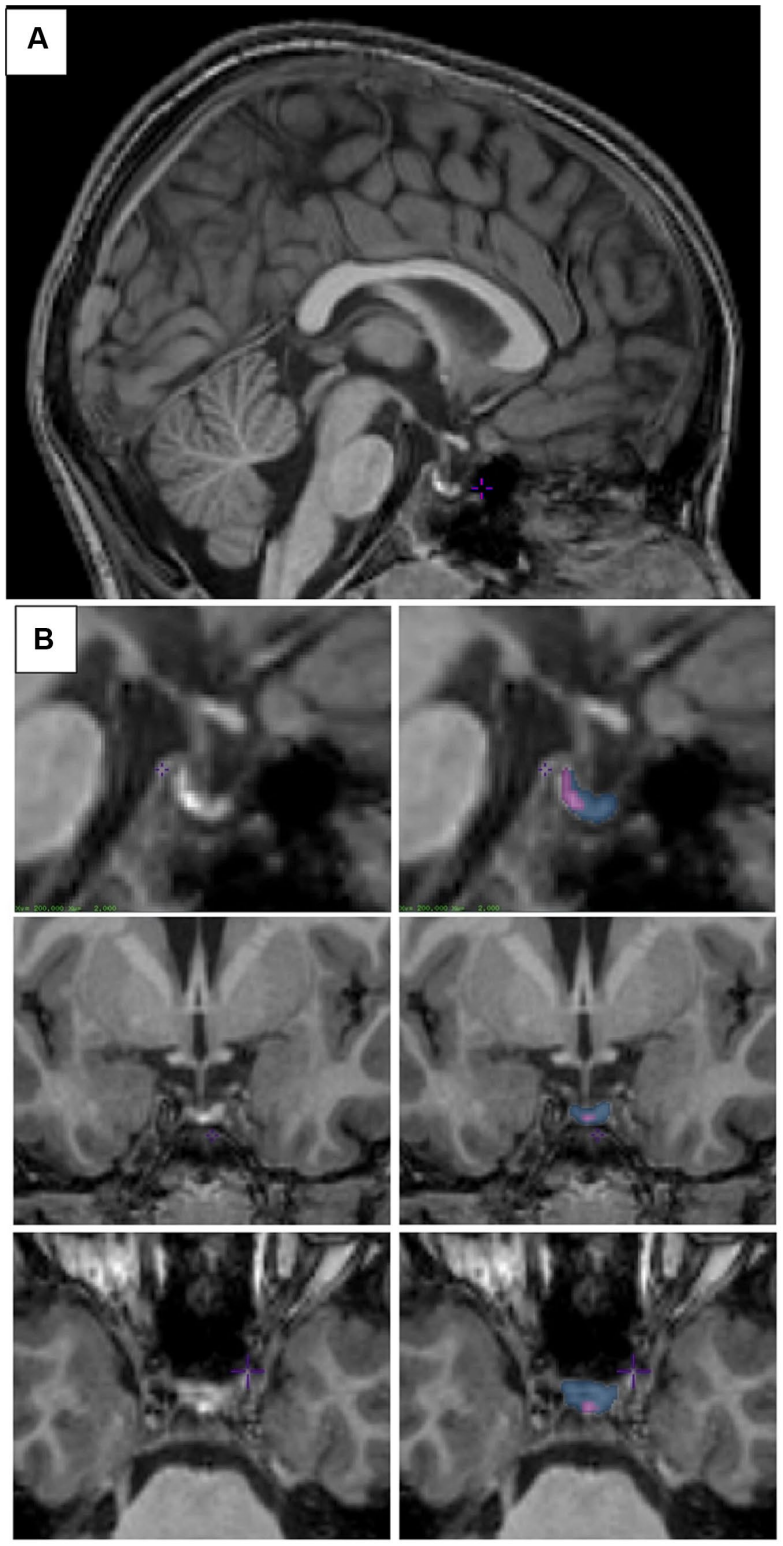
Pituitary segmentation. (A) Midsagittal view of the pituitary gland from a T1-weighted MRI image of a 6–8-year-old child. (B) Sagittal (top), coronal (middle), and axial (bottom) views of the pituitary gland from a T1-weighted MRI image. Left column shows unsegmented image, right column shows the posterior pituitary labeled in purple and the anterior pituitary labeled in blue. Images were viewed and segmented in Display (minctools, MNI).
